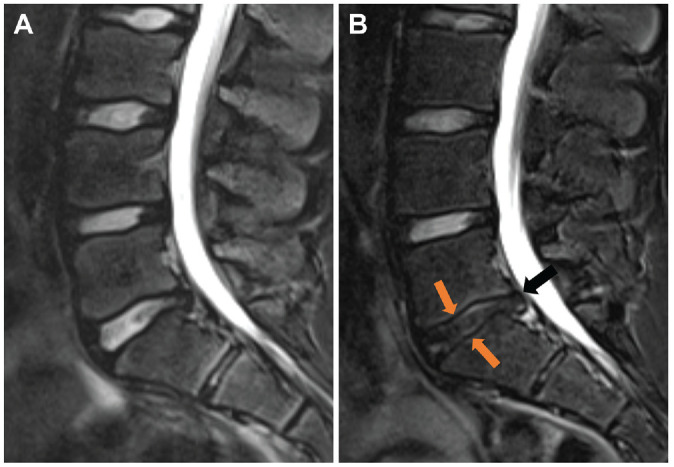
Sagittal Dixon T2-weighted magnetic resonance imaging scans of the lumbar spine of a 19-year-old competitive alpine skier (A) at baseline and (B) after 48 months. Note that in the follow-up image, the excessive progression of disk degeneration resulted in advanced signal reduction (lighter arrows) and progression of disk bulging with a new tear of the annulus fibrosus (black arrow).
